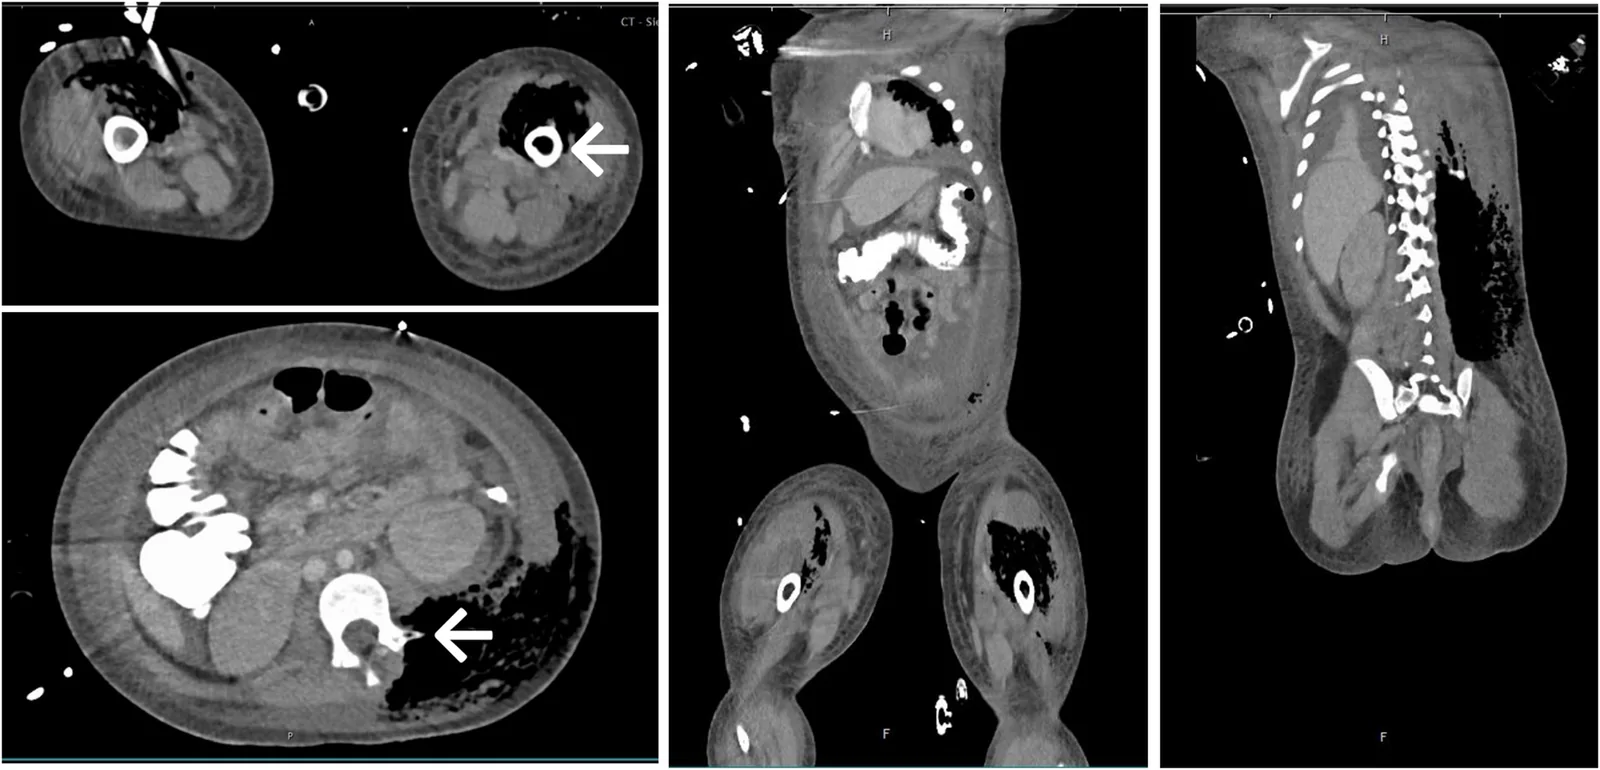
Contrast-enhanced CT scan upon admission to our institution. Extensive subcutaneous and intramuscular gas is visible in the left thoracic wall, posterior trunk, and bilateral thighs. Intraosseous gas inclusions are present in the femur and vertebral bodies (arrows). Additionally, marked thickening of the colonic wall and ascites indicate intraabdominal extension. These findings are consistent with clostridial myonecrosis with hematogenous dissemination and multi-compartimental involvement
